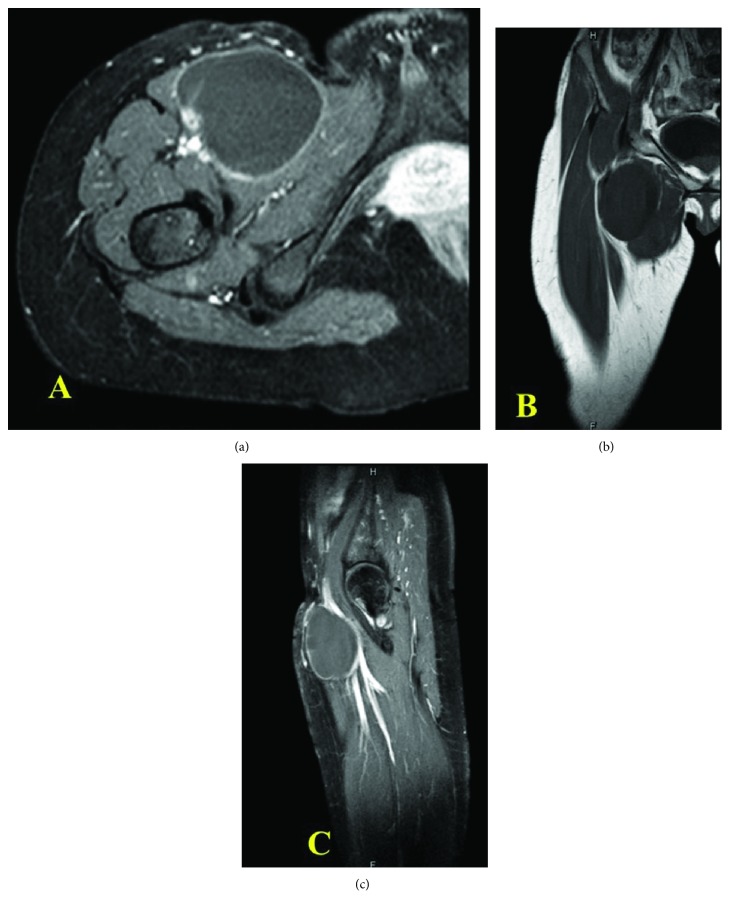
MRI shown cystic lesion: (a) axial view, (b) coronal view, and (c) sagittal view.
